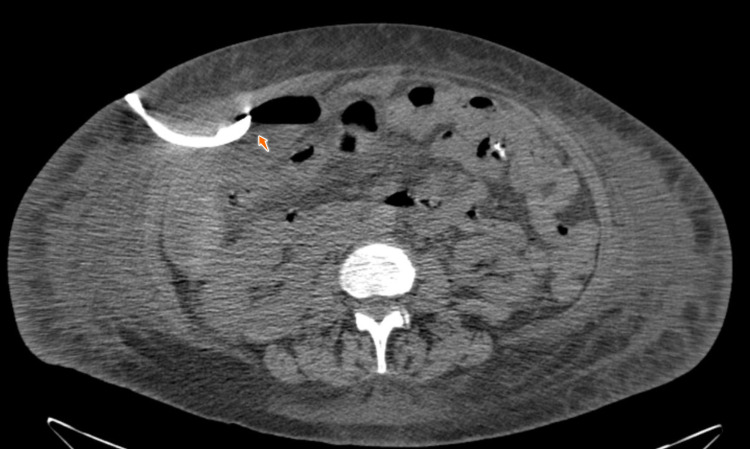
CT abdomen showing the presence of catheter for abscess drainage CT: computed tomography
